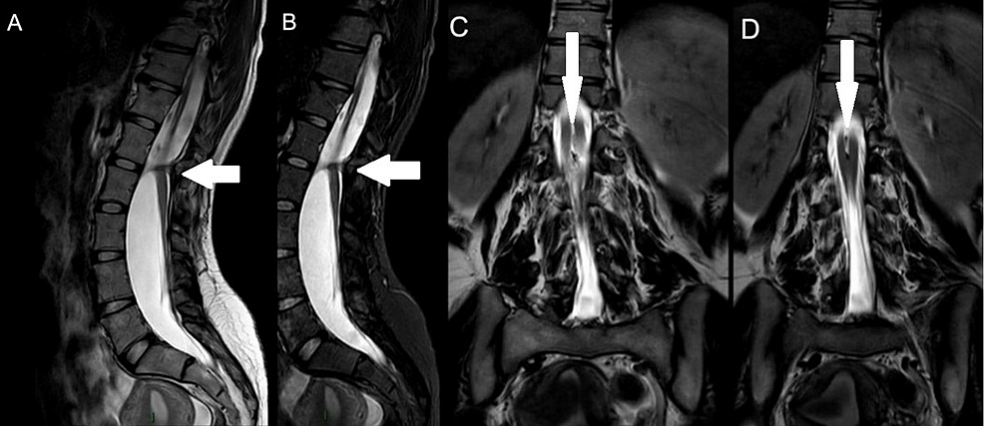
A: T2-weighted image, sagittal plane, shows the presence of a chondro-osseous ligament at the level of L1–L2. B: Short T1 inversion recovery image, sagittal plane, shows the presence of a chondro-osseous ligament at the level of L1–L2. C: T2-weighted image, coronal plane, shows the sagittal division of the spinal cord into two hemicords. D: T2-weighted image, coronal plane, shows the bifid cord joining distally.
